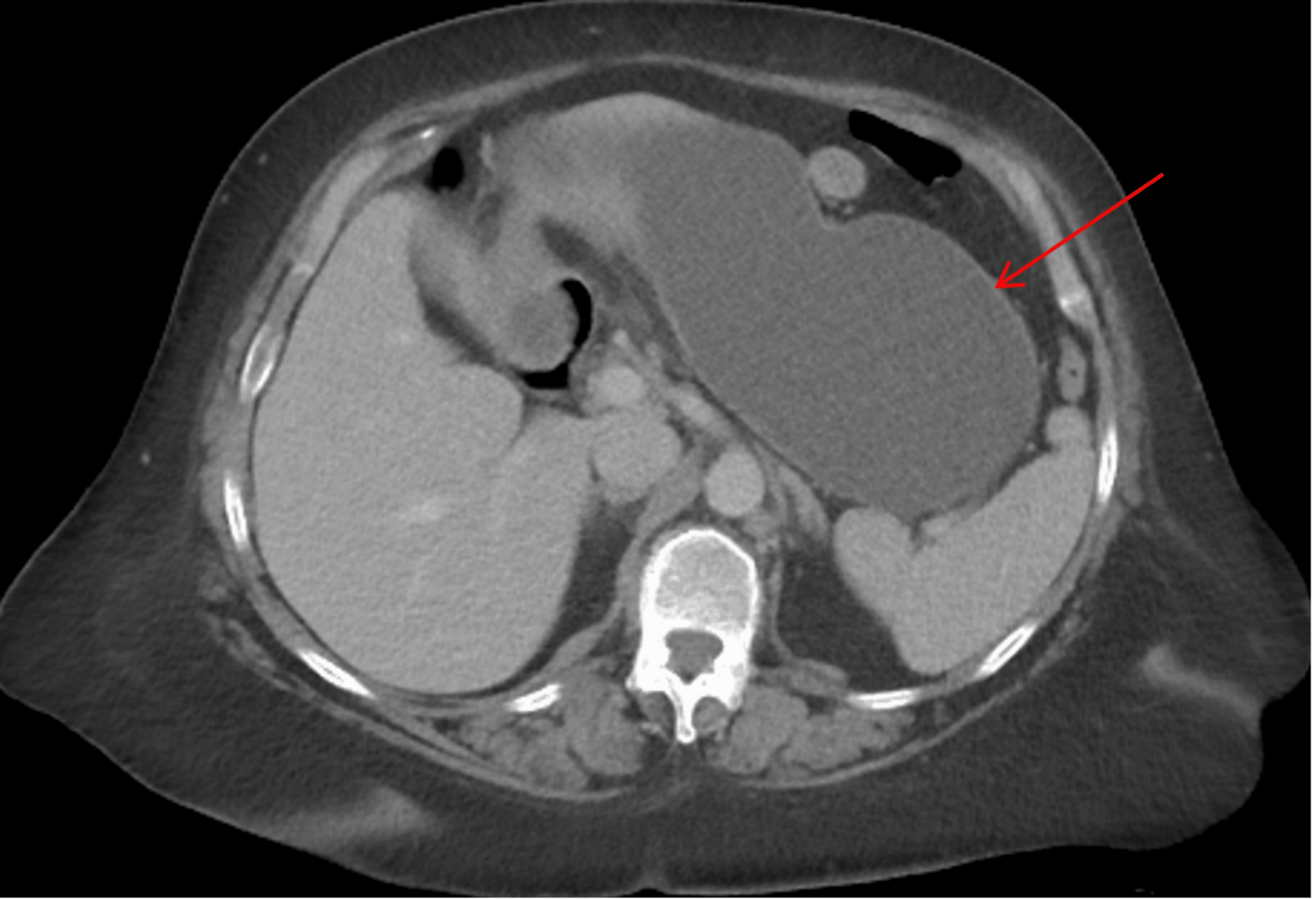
CT abdomen and pelvis with IV contrast (axial view) demonstrates a severely distended excluded stomach (red arrow)
